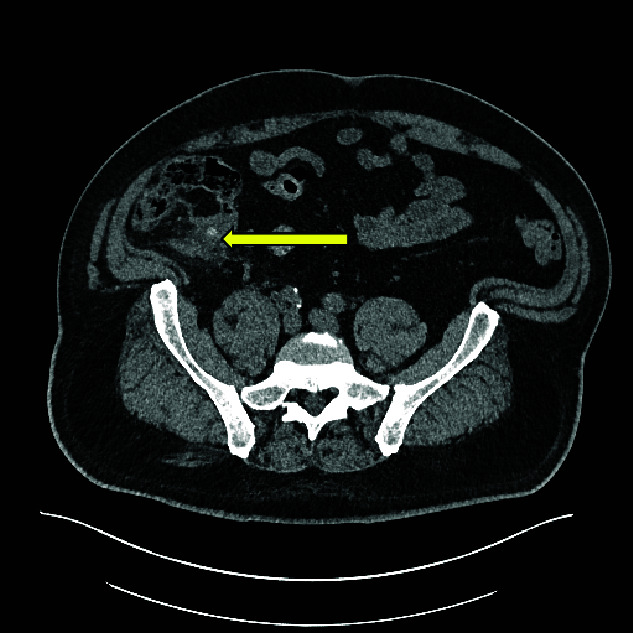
Axial CT image showing caecal inflammation and peri caecal stranding (arrow).
